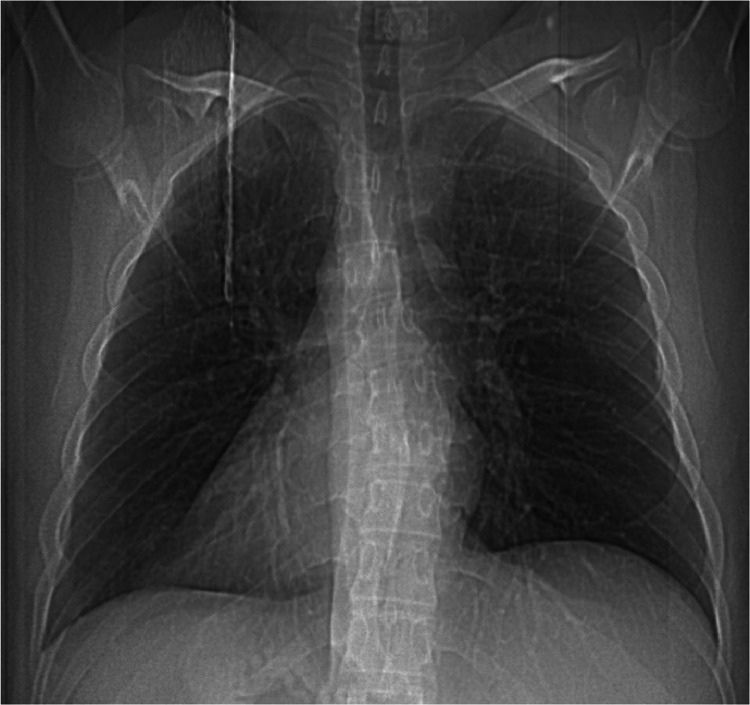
A chest X-ray showed a condition of dextrocardia and a condition of SI (Situs Inversus) in the chest. Due to this condition and the form of the rib's sinuses, a Situs Inversus Totalis (SIT) was suspected of involving the abdomen organs.
